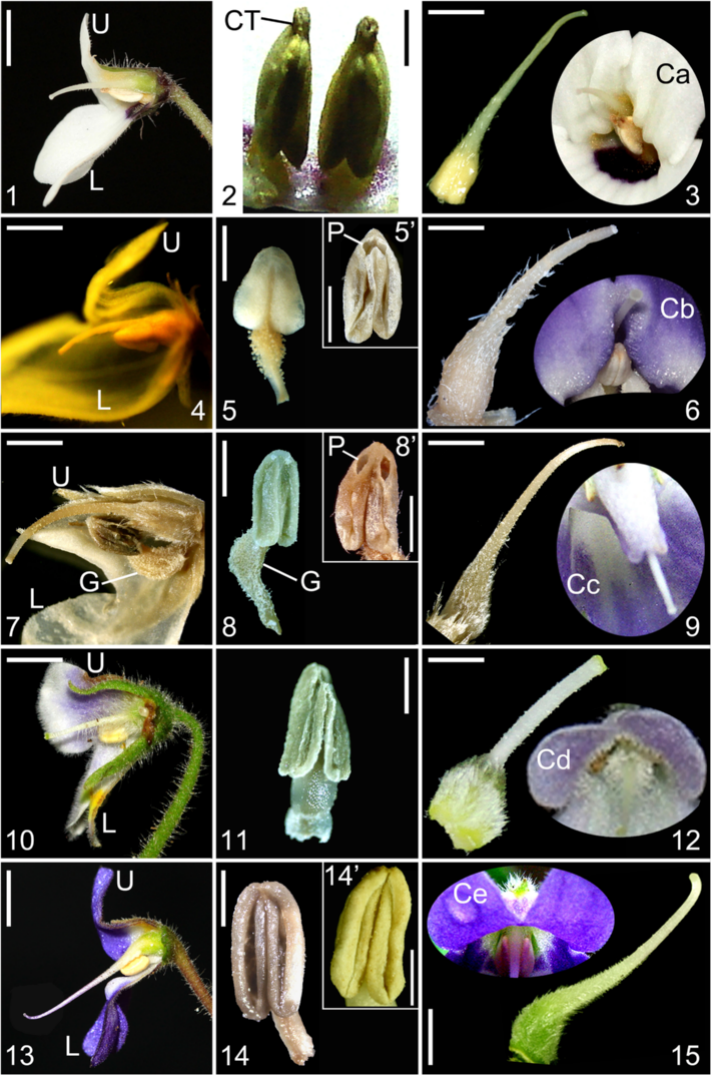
Photos of dissected flowers of representative species of different clades. 1-3 (clade A, *P. grandifolia*): 1.longitudinal section, showing relative position of stamen and pistil inside corolla tube, 2. anthers, showing anthers constricted at top, poricidal with short filament, 3. pistil, showing style’s tip curving downward, Ca showing corolla throat ribbed at both upper and lower sides and relative position of style at throat magnified 1.7 times in size relative to Fig. 1-2. Scale bars = 2.7 mm (1), 1.4 mm (2) and 2.2 mm (3) (3.5 mm in Ca). 4-6 (clade B, *P. mairei var. intraglabra*): 4. longitudinal section, 5. Stamen, showing poricidal anther basifixed with straight filament (5’. anther of *P. coerulea* showing dehiscent pore), 6. Pistil, Cb showing corolla throat of *P. coerulea* ribbed at upper side and relative position of style at throat magnified 2.5 times in size relative to Fig. 1-8. Scale bars = 3.4 mm (4), 1.1 mm (5) (0.86 mm in 5’) and 1.3 mm (6) (2 mm in Cb). 7-9 (clade C, *P. sericea*): 7. longitudinal section, 8. Stamen, showing poricidal anther basifixed with long geniculate filament (8’. anther of *P. minor* showing dehiscent pore), 9. pistil, showing style curving downward at top, Cc showing corolla throat unribbed and relative position of style at throat magnified 2 times in size relative to Fig. 1-13. Scale bars = 2.9 mm (7), 1.8 mm (8) (1.6 mm in 8’) and 2.2 mm (9) (2.1 mm in Cc). 10-12 (clade D, *P. forrestii* and *P. barbata*): 10. longitudinal section of *P. barbata*, showing style extending from centre of the throat, 11. Stamen of *P. forrestii*, showing anther longitudinal dehiscent with short filament, 12. Pistil of *P. forrestii*, erect, Cd showing corolla throat of *P. barbata* unribbed and relative position of style at throat magnified 1.75 times in size relative to Fig. 1-17. Scale bars = 3.6 mm (10), 1.3 mm (11) and 1.9 mm (12) (2.6 mm in Cd). 13-15 (clade E, *P. sinensis*): 13. longitudinal section, 14. Stamen, showing longitudinal anther with short filament (14’. showing anther with longitudinal dehiscence becoming visible), 15. pistil, showing style curving downward at the base and curving upward at the top, Ce showing corolla throat unribbed and relative position of style at throat magnified 1.26 times in size relative to Fig. 1-24. Scale bars = 3.7 mm (13), 1.4 mm (14 and 14’) and 2.9 mm (15) (4.2 mm in Ce). CT, constriction; G, geniculate; L, lower lip; P. dehiscent pore; U, upper lip
